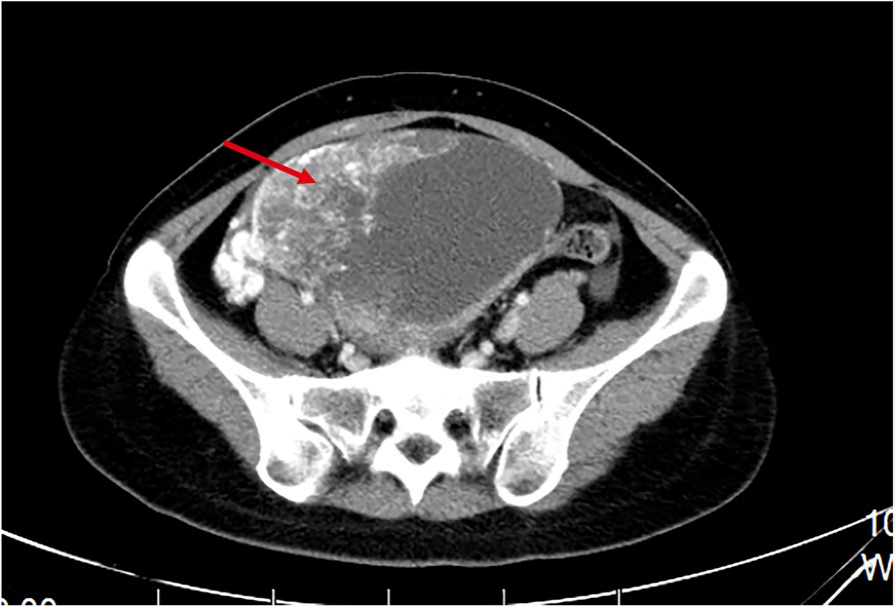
Computed tomography (CT) revealed a 14 cm solid cystic mass in the right adnexa
